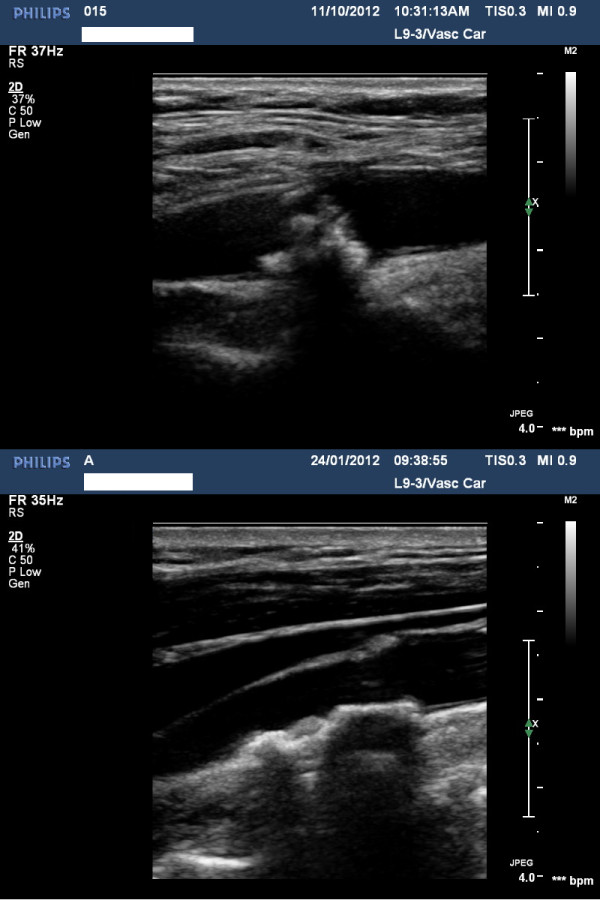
Full-size ultrasound images corresponding to the close-up plaque views shown in Figure 1. (top row) the symptomatic plaque, (bottom row) the asymptomatic plaque.
